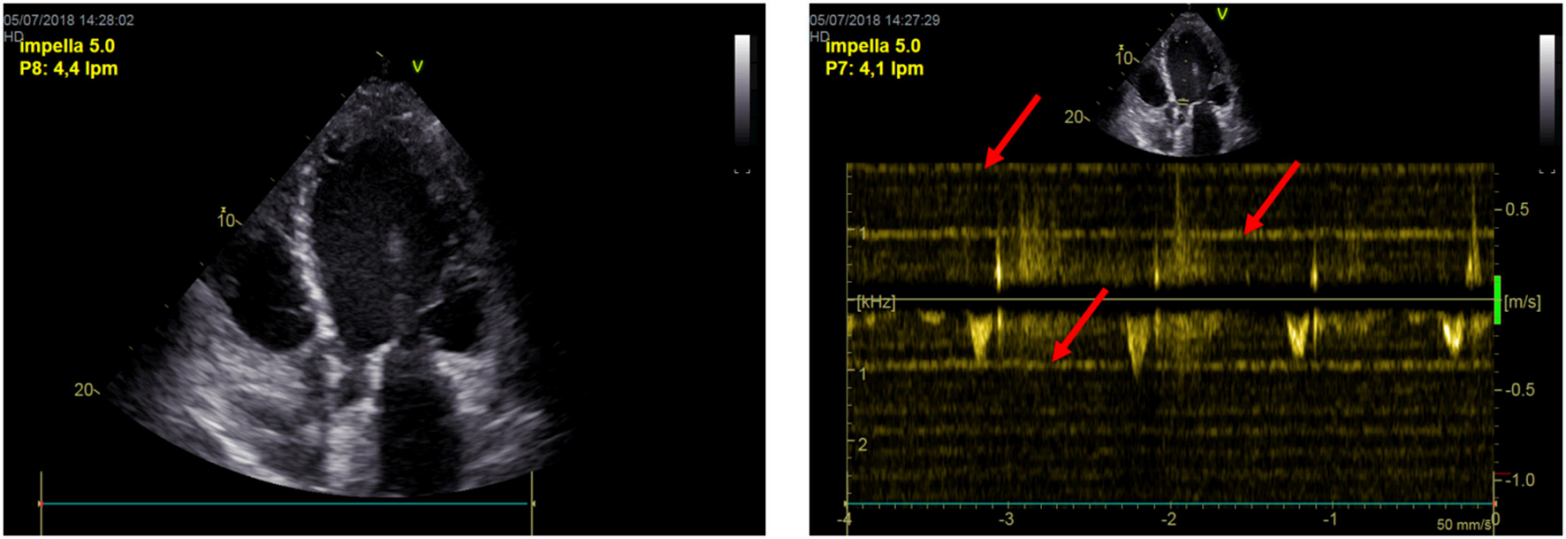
Impella's artifacts interfere in 2-dimensional echo and Doppler images of the heart.
